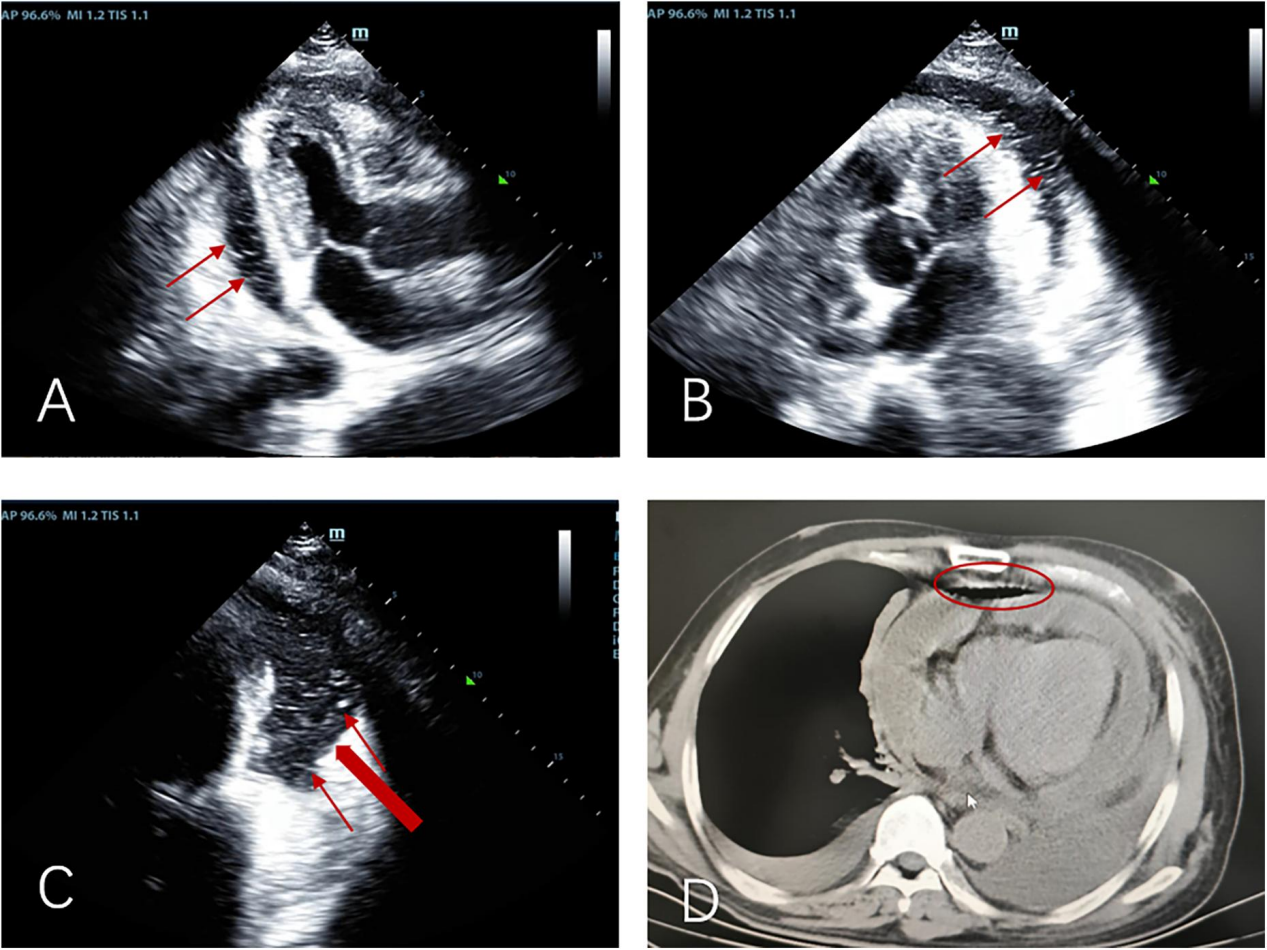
Apical three-chamber view (A), apical five-chamber view (B), and apical four-chamber view (C) consistently demonstrate: moderate pericardial effusion with pneumopericardium, “Swirling microbubble sign” (microbubbles originating from the lateral pericardium, thin red arrow), suspected communication between pericardial space and bronchus/residual lung tissue (thick red arrow); (D) pre-decompression chest CT demonstrating gas and effusion accumulation in the pericardial cavity.
